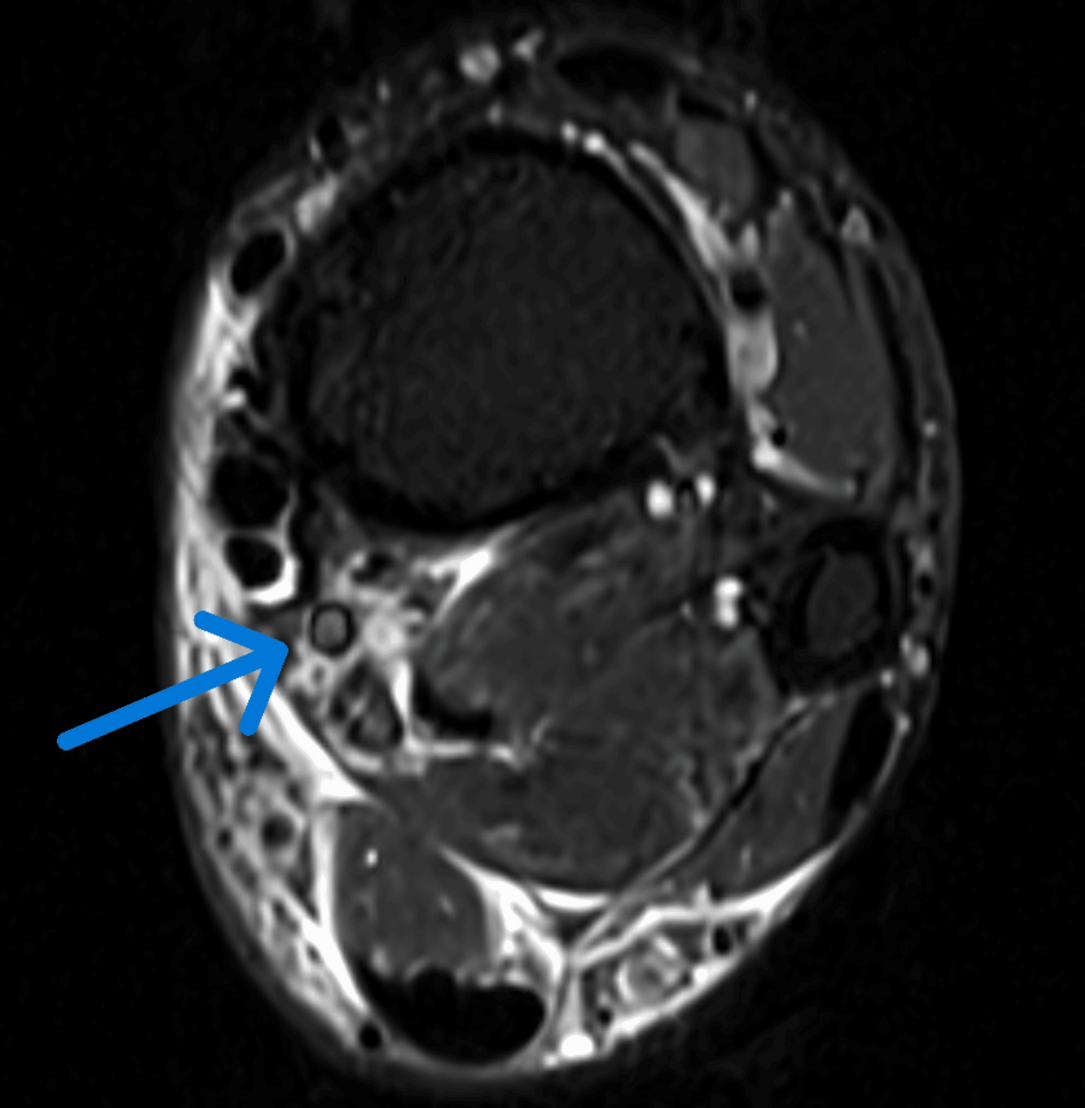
MRI revealing vein thrombosis in the distal leg Vein thrombosis in the distal leg, at the level of the distal tibia and fibula, just above the ankle.
